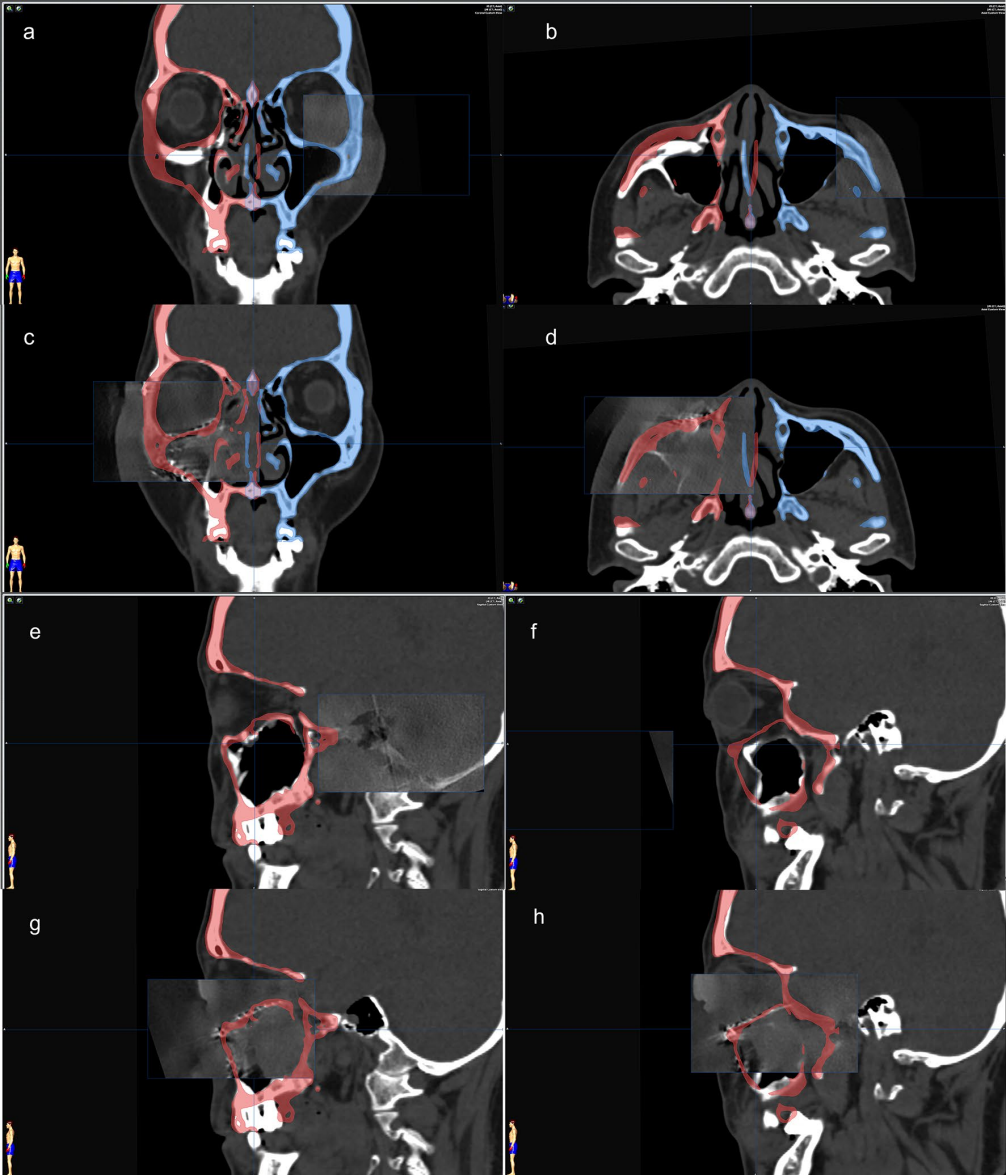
Fusion of preoperative computed tomography image, navigation presurgical planning, and intraoperative 3D C-arm computed tomography image. The intraoperative 3D C-arm computed tomography image is merged partially to the objects of navigation planning at the unaffected side (left) on the coronal and axial view (a,b). The updated position of the reduced zygoma and reconstructed orbital floor with implants (c,d). From different panels of the sagittal view, the preoperative images (e,f) and intraoperative images after implant positioning (g,h). The bone and implant contour accurately matched the presurgical planning.
